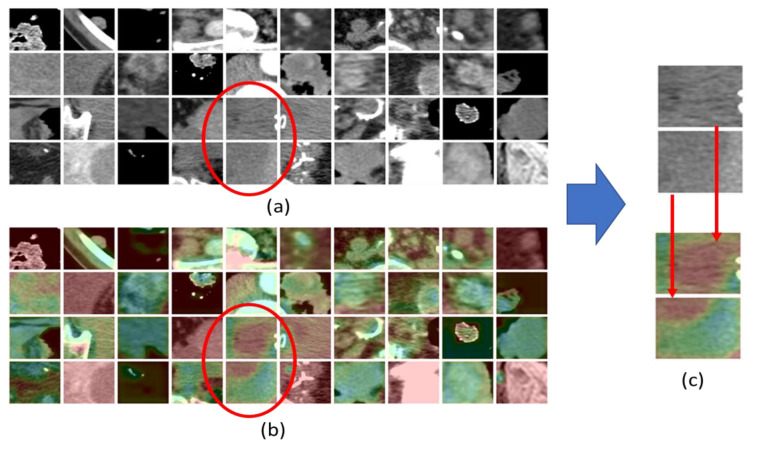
(a) RECIST (Response Evaluation Criteria in Solid Tumors) 1.1 compliant CT target lesions. (b) The corresponding Grad-CAM generated saliency maps. (c) A detail of the salient part of the processed RECIST lesion.
